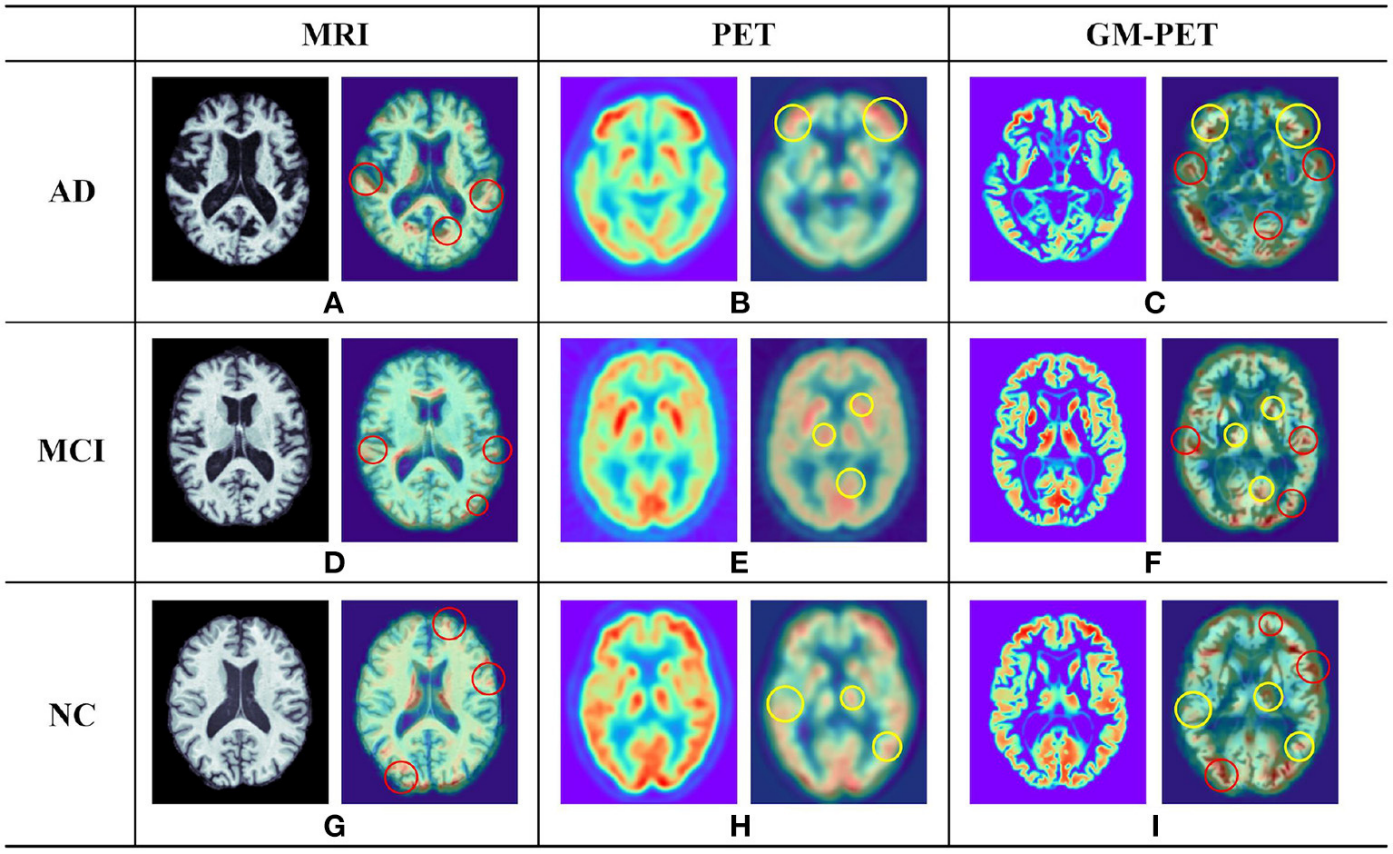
Examples of different modality images for AD, MCI, and NC subjects. In each of the nine cells (A–I), the picture on the left is a subject slice and the picture on the right is the Grad-CAM result for that slice. The red circle in the 3D Grad-CAM results outlines the contour areas of common interest in the MRI and GM-PET images, while the yellow circle outlines the metabolic characteristic areas of common interest in the PET and GM-PET images.
